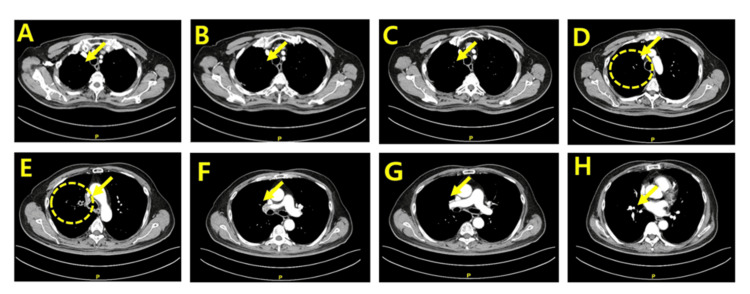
Post-treatment axial CT images after five cycles of therapy (2025-10-09, Slices A–H) Matched axial CT slices (A–H) obtained after completion of five cycles of platinum–etoposide–atezolizumab therapy. Across all levels, the previously identified mediastinal mass has regressed almost completely, with restoration of normal mediastinal contour, re-expansion of adjacent lung parenchyma, and absence of any mass-like density. Residual fibrosis is minimal, and no new intrathoracic lesions are detected. These images demonstrate a near-complete structural response to systemic therapy. Arrows indicate the site of resected small cell lung cancer.
